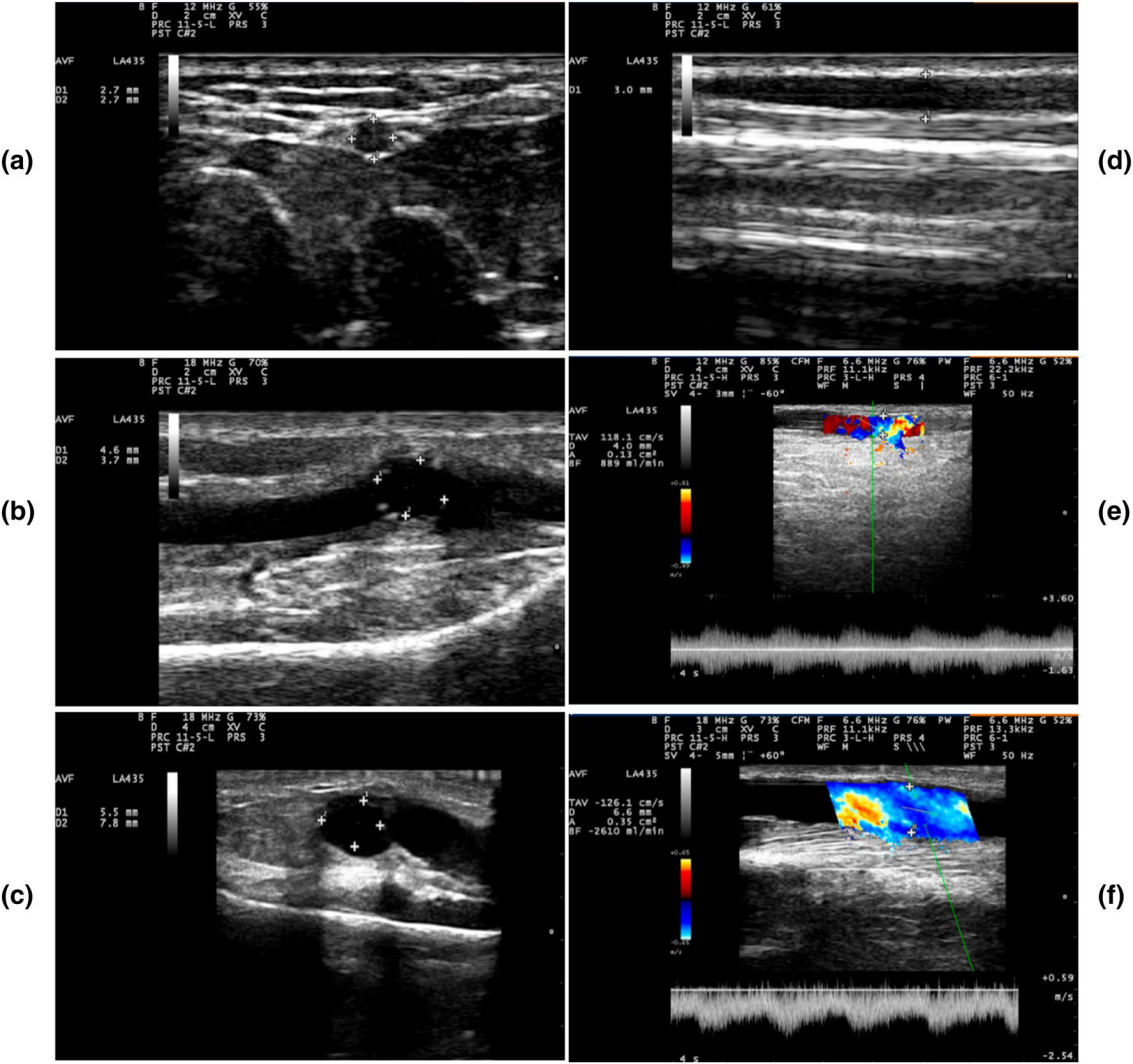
The diameter and the blood flow of vessels in MLPN group under ultrasound examination: a DA (2.7*2.7 mm) at T0, b DA (4.6*3.7 mm) at T2, c DA (5.5*7.8 mm) at T5, d DV (3.0 mm) at T0, e DV (4.0 mm) and BFV (889 ml/min) at T0, f DV (6.6 mm) and BFV (2610 ml/min) at T5. MLPN: moxibustion at Laogong interval with *Panax notoginseng*; DA: diameter of anastomosis; DV: diameter of draining-veins; BFV: blood flow of draining-veins
